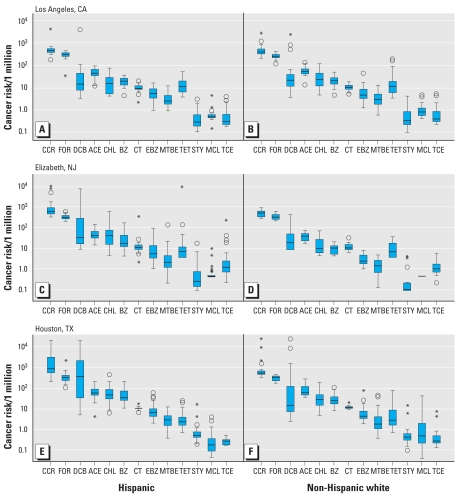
Distributions of cancer risks based on personal concentrations of Hispanics and whites in Los Angeles (*A* and *B*), Elizabeth (*C* and *D*), and Houston (*E* and *F*). Boxes represent 25th and 75th percentiles, whiskers indicate lower and upper range, and the black line is the median. Circles indicate values between 1.5 and 3 times the interquartile range. Asterisks demonstrate values > 3 times the interquartile range. Hispanic: Los Angeles, *n* = 23; Elizabeth, *n* = 54; Houston, *n* = 44. Non-Hispanic white: Los Angeles, *n* = 43; Elizabeth, *n* = 15; Houston, *n* = 36. Abbreviations: ACE, acetaldehyde; BZ, benzene; CCR, cumulative cancer risk; CHL, chloroform; CT, carbon tetrachloride; DCB, p-dichlorobenzene; EBZ, ethylbenzene; FOR, formaldehyde; MCL, methylene chloride; MTBE, methyl tert-butyl ether; STY, styrene; TCE, trichloroethylene; TET, tetrachloroethylene.
